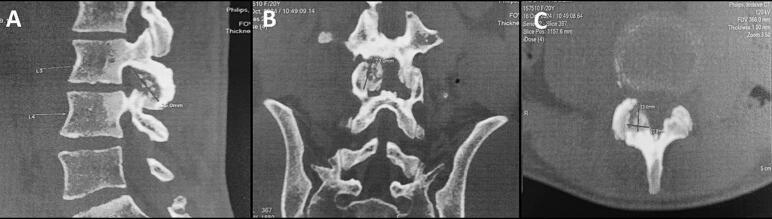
(A, B, C): CT scan showing a well-defined lytic lesion located at the right inferior articular process of L3, extending to the inferior articular surface of L3 and the adjacent superior articular process of L4. The lesion includes a calcified nidus causing cortical disruption, surrounded by osteocondensation with blurred borders.
